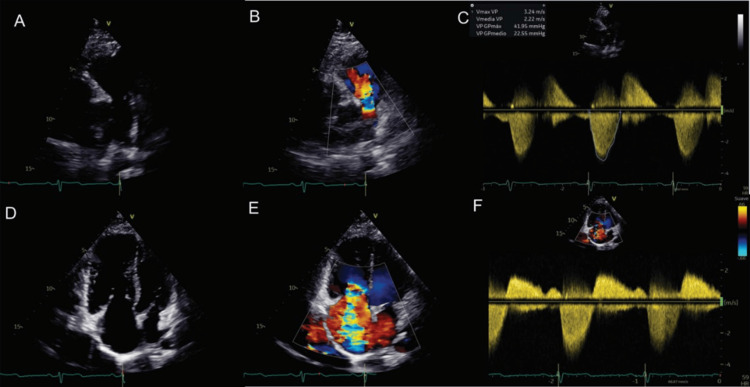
Transthoracic echocardiography A) A parasternal short axis view focused on the pulmonary valve shows the thickening of the cusps and limitation of their mobility. B) Color Doppler echocardiography displays a wide jet of pulmonary regurgitation (PR). C) Evaluation of the pulmonary valve by continuous wave Doppler compatible with moderate stenosis (peak velocity 3.2 m/s, peak gradient 41 mmHg) and severe regurgitation (PR index of 0.65). D) An apical four-chamber right ventricle focused view demonstrates thickening of the tricuspid leaflets mainly on the tips and a wide coaptation gap in systole. D) Color Doppler echocardiography shows a severe tricuspid regurgitation (TR) jet. E) Evaluation by continuous wave Doppler. A dense triangulated regurgitant jet consistent with severe TR is observed; the mean pressure gradient (4 mmHg) and the pressure half-time (173 milliseconds) were consistent with moderate tricuspid stenosis.
